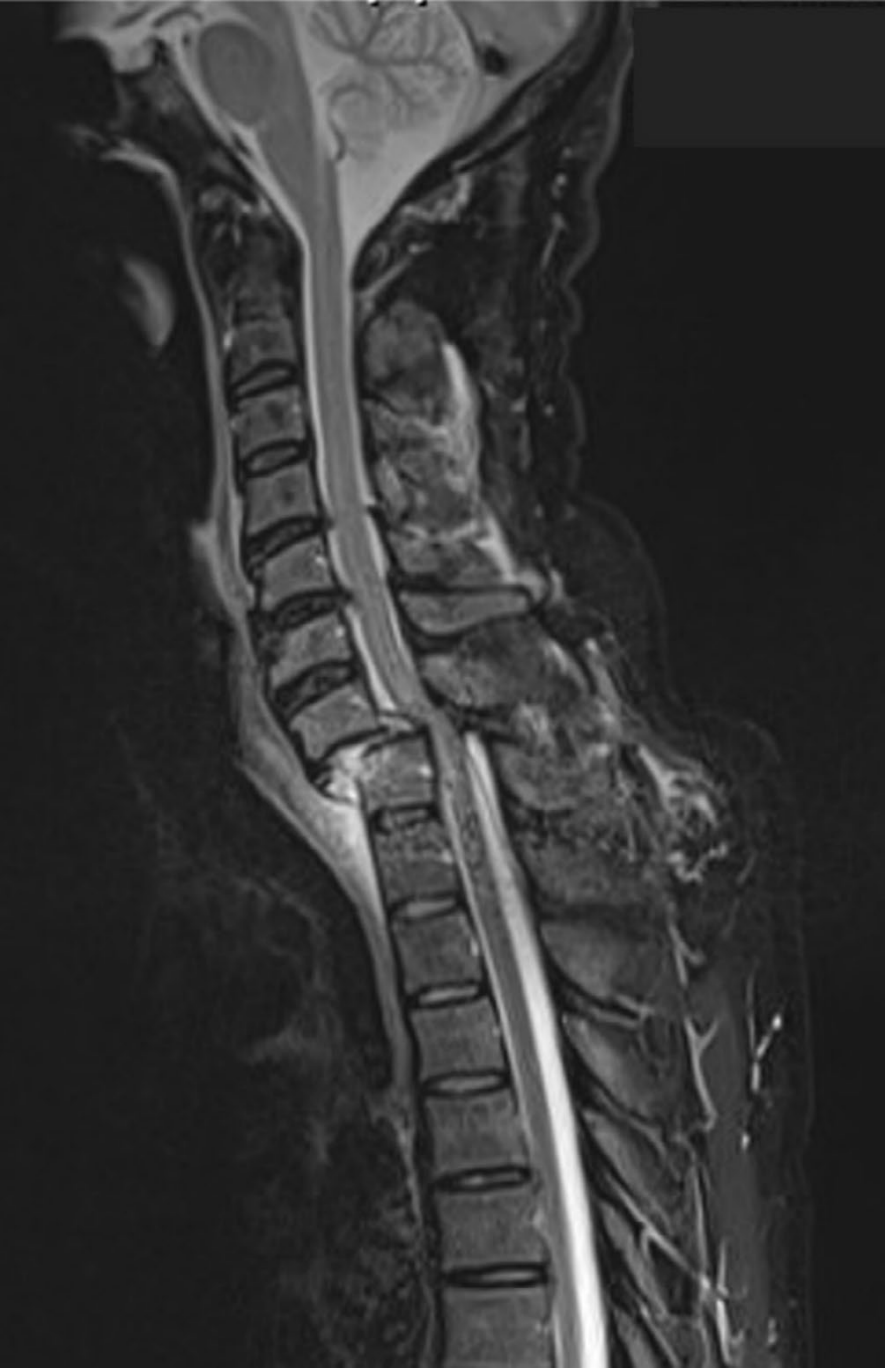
Magnetic resonance imaging of the cervical and upper thoracic spine of the case report. Dislocation of cervical vertebral body 7 against thoracic vertebral body 1 with resulting spinal canal stenosis and severe spinal cord compression.
